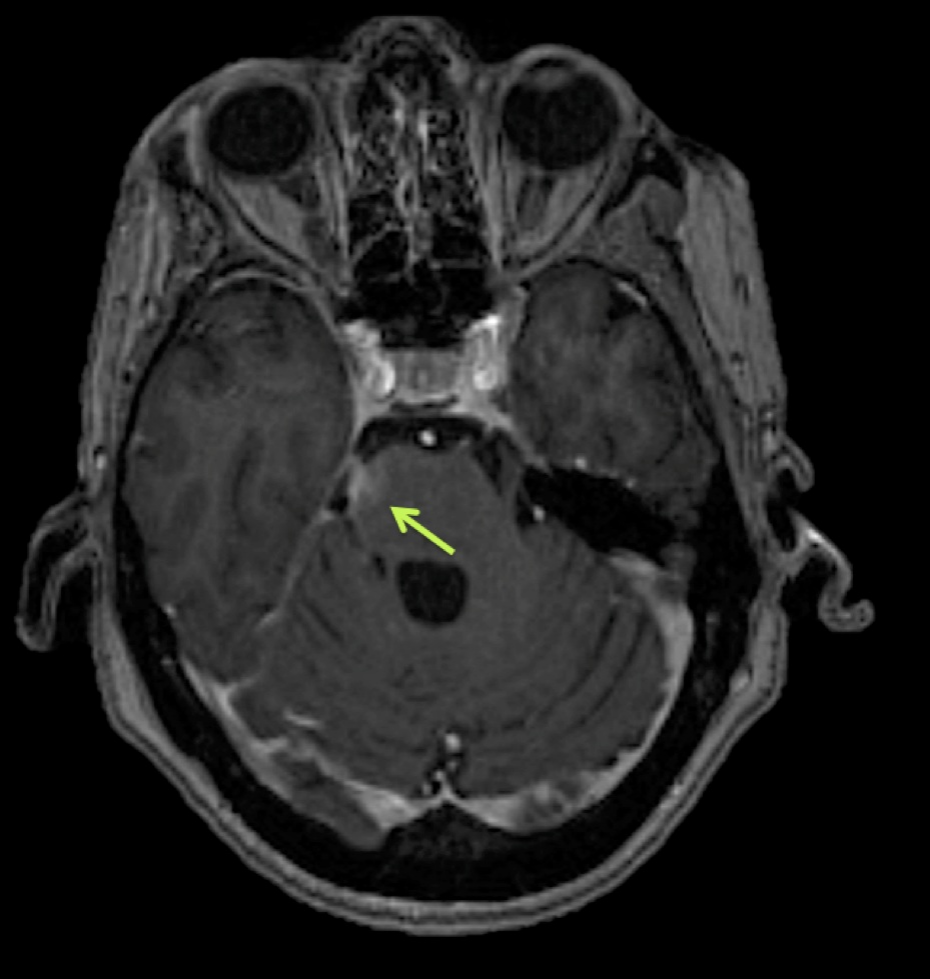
Brain MRI Post-Gadolinium axial T1-weighted image showing a right paramedian pontine area of contrast enhancement, at the level of the emergence of the fifth cranial nerve (green arrow).
